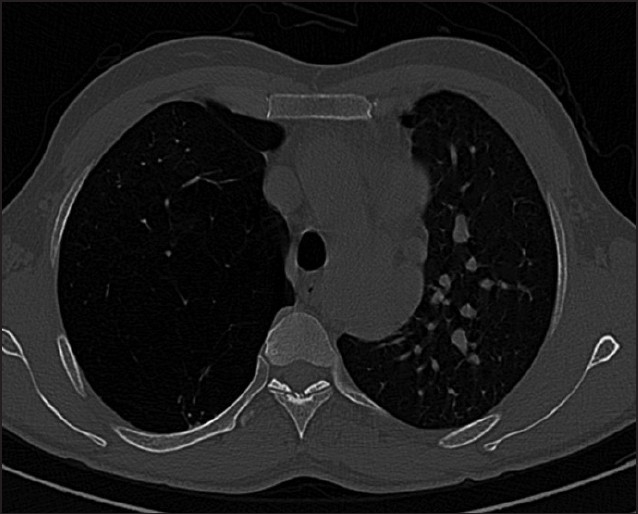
High-resolution CT shows emphysematous right upper lobe with attenuated vascular markings; the accessory hemiazygous is seen draining into the left superior vena cava.
